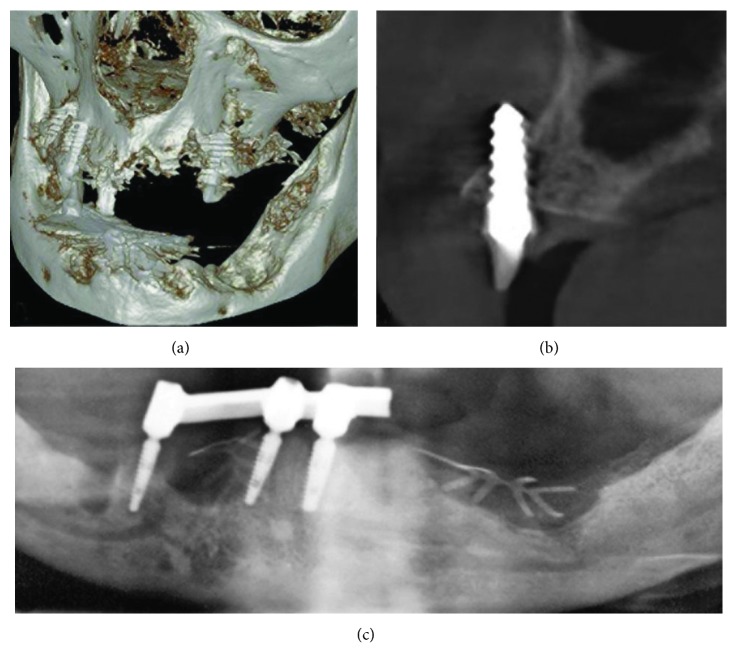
(a) Volumetric 3D representation of hard tissue and maxillary implants, taken during mandibular surgery, demonstrating large bone defects and loss of buccal bone plate in the maxilla. (b) Axial CBCT section of the maxilla showing misplaced implant #4. (c) OPG section showing bar retained on the remaining three mandibular implants (not modified radiograph taken during current treatment).
